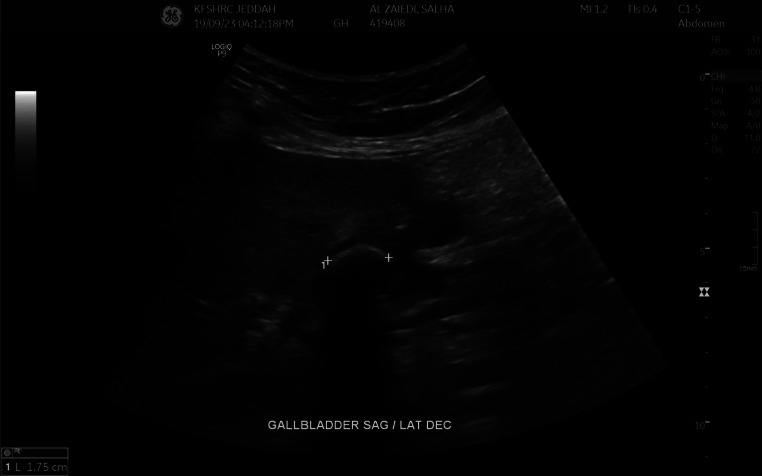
Grayscale ultrasound image. The image shows a large hyperechoic stone measuring 1.75 cm, showing posterior acoustic shadowing (white arrow); the stone was not visible on CT scan.
